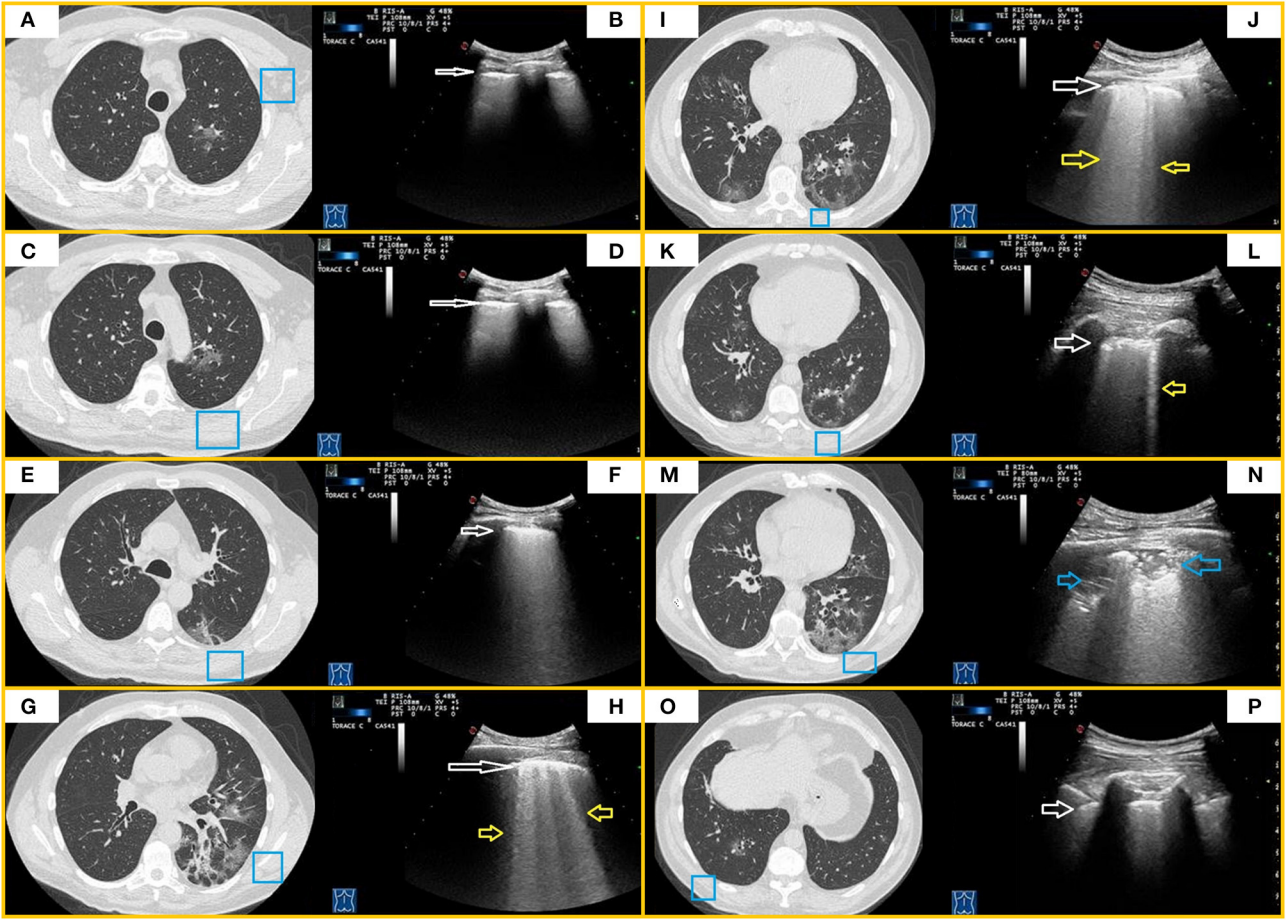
CT and TUS findings in moderate COVID-19 pneumonia. A 67-year-old male patient presenting in ED with fever and cough for 10 days. The RT-PCR assay on nasopharyngeal swab confirmed the suspect for COVID-19 pneumonia. In (A,C), axial CT scans passing through the upper lobes show central areas of pure ground glass in the left upper lobe. In (E), axial CT scan passing through the carina level shows a peripheral area of pure ground glass in the left superior lower lobe. In (B,D,F), ultrasound scans with a convex probe (6 MHz) and thoracic setting, corresponding to the blue boxes on the respective CT scans, show a thickened hyperechoic pleural line without B-lines (white arrow). In (G,I,K), axial CT scans passing through middle-lower zones show multiple confluent areas of ground glass opacities, with peri-bronchovascular and subpleural distribution, in the lingula and right and left lower lobes. Associated linear opacities with peri-lobular pattern and bronchiectasis are observable in left lower lobes. Initial bronchiectasis is visible in the middle lobe. In (H,J,L), ultrasound scans with a convex probe (6 MHz) and thoracic setting, corresponding to the blue boxes in the respective CT scans, show an irregular and thickened hyperechoic pleural line (white arrow) with focal or coalescent B-lines (yellow arrows). In (M), axial CT scan passing through the basal lung regions shows multiple subpleural patchy areas of pure ground glass opacities adherent to pleural surface in the posterior region of the left lower lobe. In (N), ultrasound scan with a convex probe (6 MHz) and thoracic setting, corresponding to the blue box in the respective CT scan, shows mixed hypoechoic subpleural irregular consolidation (blue arrow). In (O), axial CT scan passing through the basal lung regions shows some pure ground glass opacities, the largest of which is localized in the right lower lobe along the bronchovascular structures. In (P), ultrasound scan with a convex probe (6 MHz) and thoracic setting, corresponding to the blue box in the respective CT scans, shows a thickened hyperechoic pleural line (white arrow) without B-lines.
